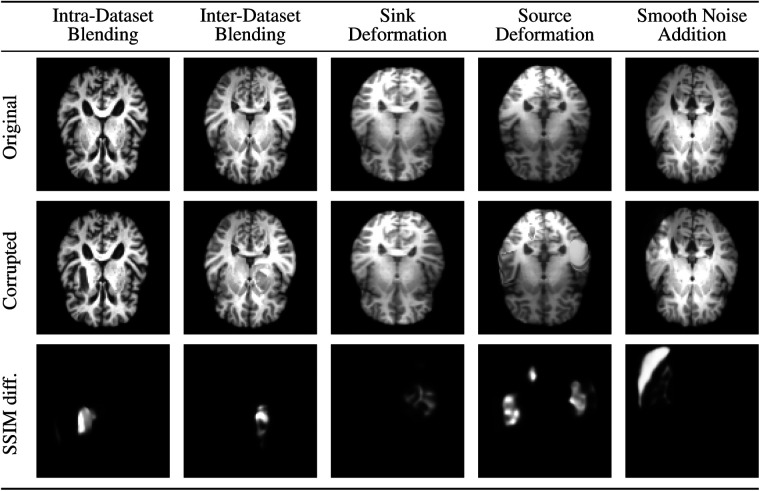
Examples of each of the synthetic anomaly tasks applied to T1-weighted brain MRI. Each task is applied to the full 3D volume up to 3 times although we only visualise the central axial slice here.
